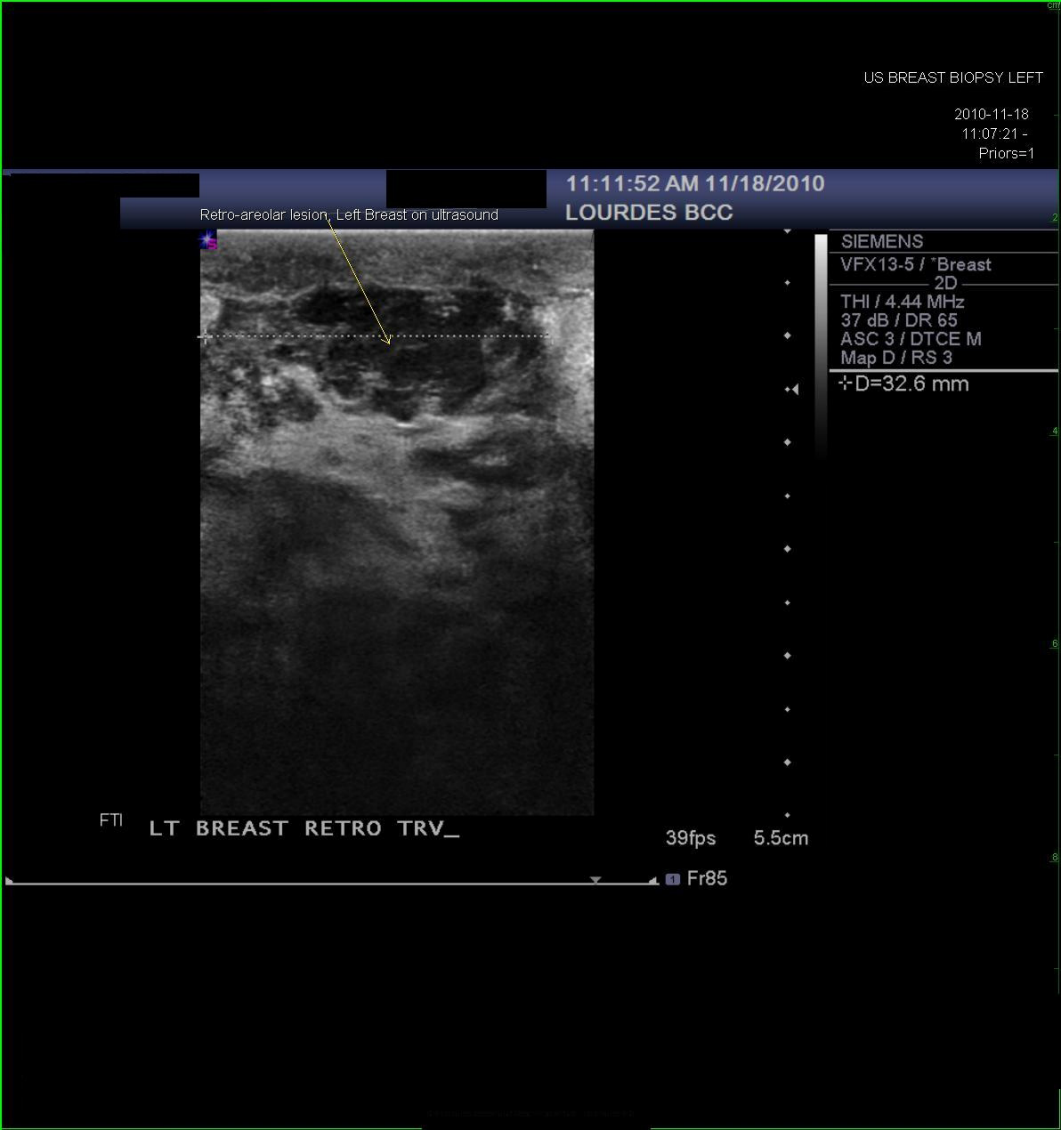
An ultrasound image of the left breast. An ultrasound image of the left breast, retro-areolar location, which reveals an ill-defined, hypo-echoic, heterogenous mass corresponding to the palpable mass on physical exam and the larger mass on the CAT scan of the chest.
